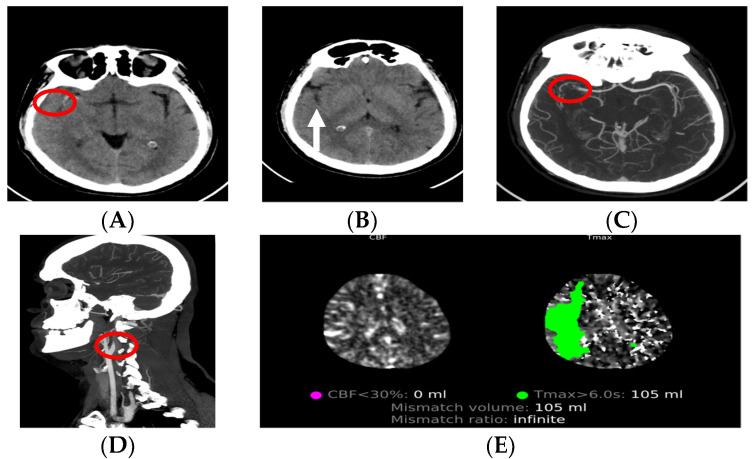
Patient, male, 48 years old, presented with left hemiplegia and dysarthria at the emergency department, after which a neurologist ordered CT diagnostics. (A,B) Non-contrast CT which shows MCA hyperdensity (red circle), loss of right insular ribbon (white arrow) and ASPECT 9. (C,D) CT angiography, axial and sagittal, showing tandem occlusion of origin of R-ICA (dissection) and R-MCA (both in red circles). (E) CT perfusion showing mismatch volume of 105 mL.
